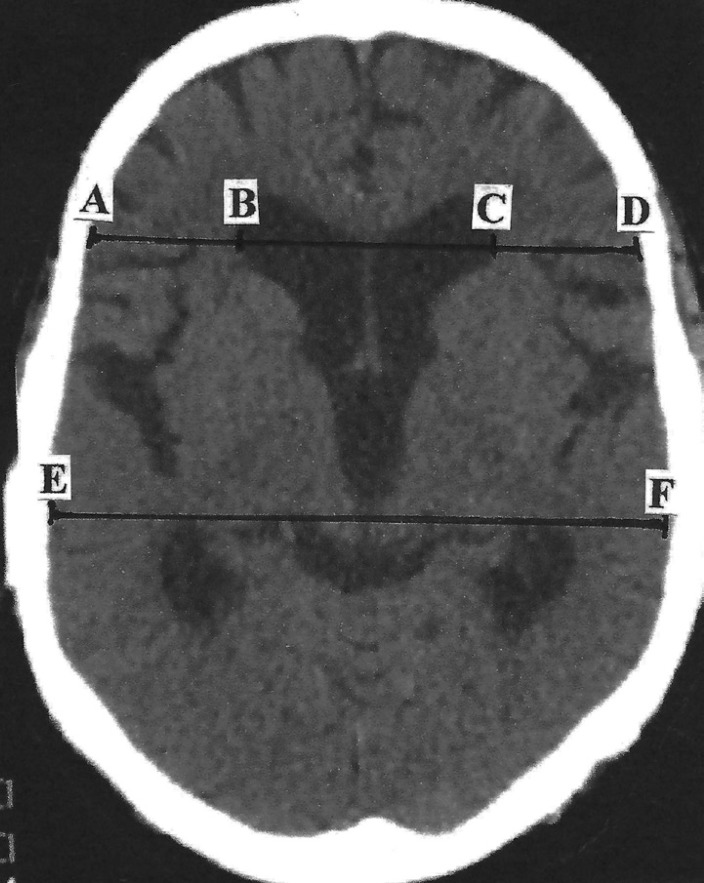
Axial CT slice of the brain in a patient with NPH. The Evans index can be
